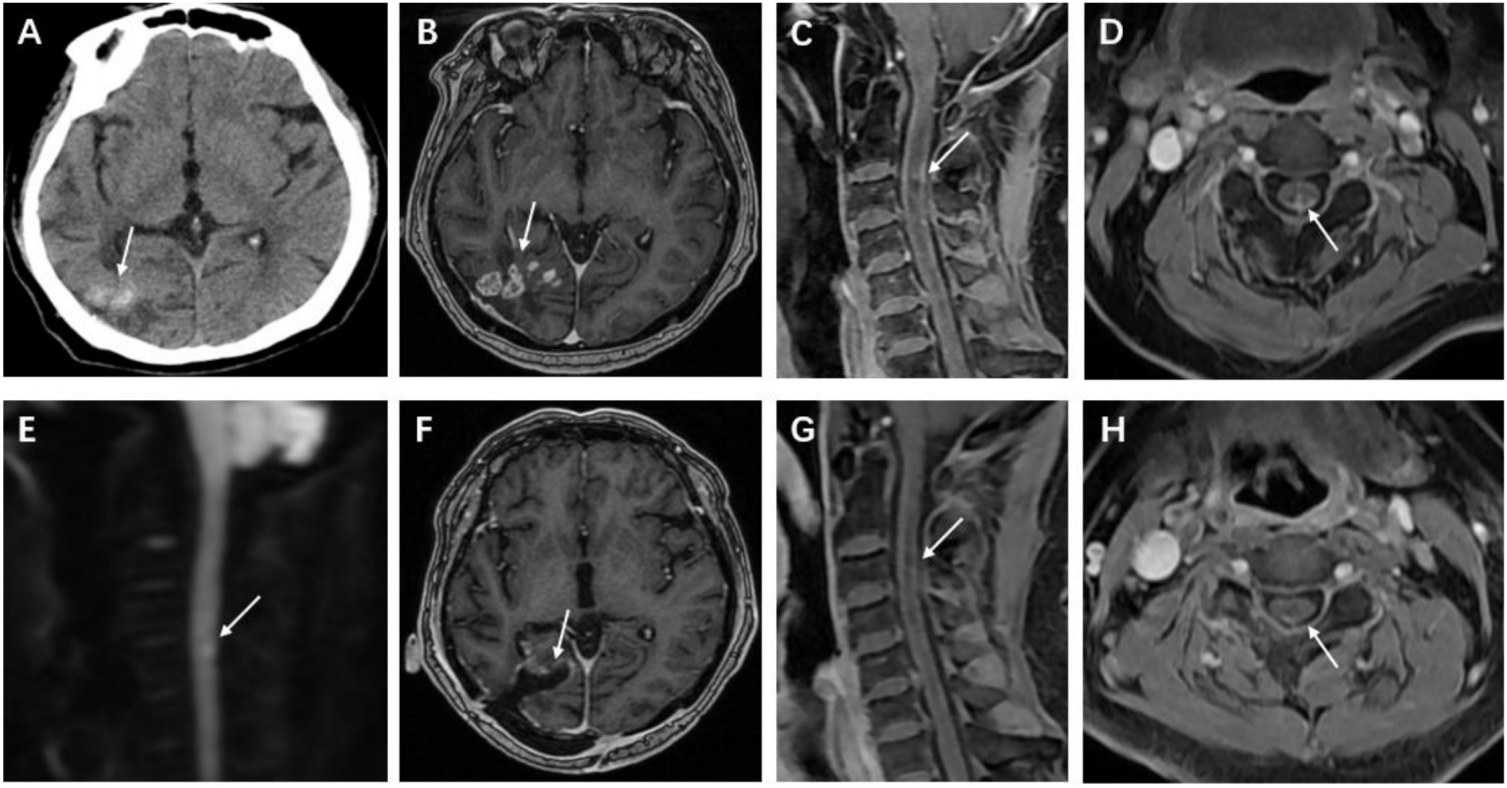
Cranial CT and MRI after hospital admission. (A, B) Cranial computed tomography (CT) shows a right temporoparietal-occipital lobe lesion exhibiting mixed high and low densities; contrast-enhanced examination reveals multiple circularly enhancing foci within the lesion and adjacent meningeal enhancement. (C, D) Spinal magnetic resonance imaging (MRI) reveals nodular enhancing foci within the spinal cord at the C3-C5 levels, accompanied by adjacent pial enhancement. (E) Diffusion-weighted imaging (DWI) demonstrates restricted diffusion within the spinal lesions. Follow-up brain and cervical spine MRI 8 weeks after surgery. (F) Postoperative MRI of the brain demonstrates changes in the right temporo-occipital lobe, with no abnormal enhancing lesions. (G, H) Cervical spine MRI shows that the extent of abnormal enhancing lesions in the spinal cord is reduced.
